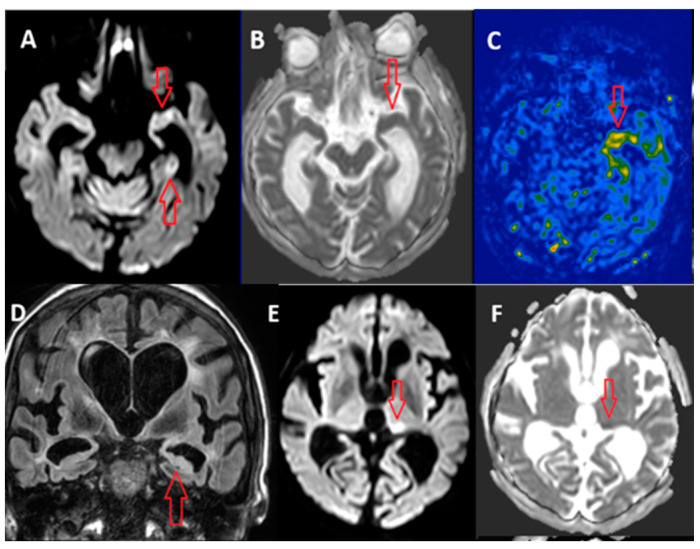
(personal courtesy): Example of PMA in the acute MRI in a patient with NCSE taken in less than 24 h after the onset of SE: diffusion restriction (A), normal ADC (B), hyper-perfusion in ASL (C) and signal increase in FLAIR (D) in the left hippocampus (red arrow). Diffusion restriction (E) and signal decrease in ADC map (F) on the left pulvinar of the thalamus (red arrow).
